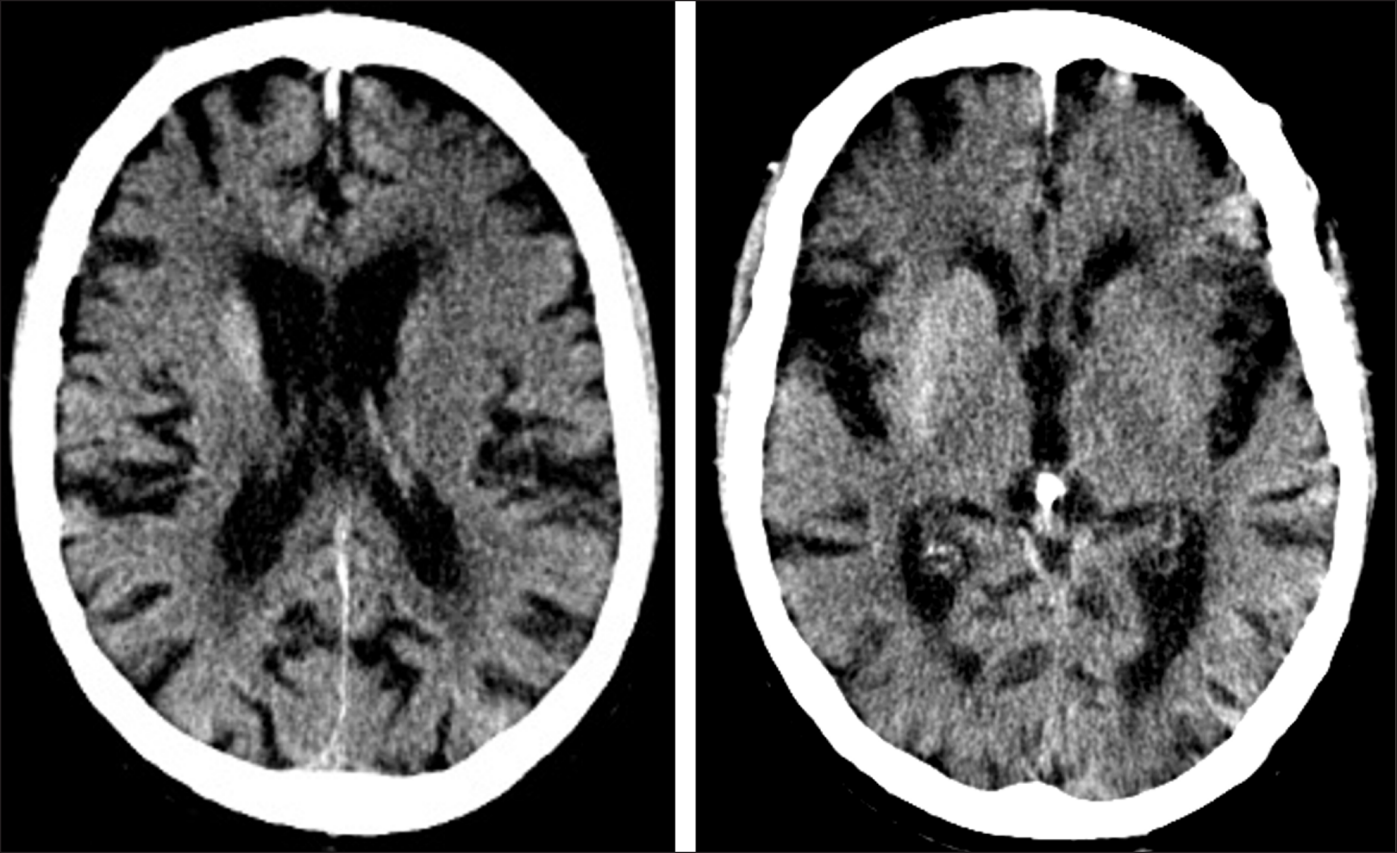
Hemoglobin A1c (HbA1c) and blood glucose level were measured. The glycemia was normal, but high HbA1c of 13.8% or 127 mmol/mol (normal 4–6% or 20–42mmol/mol) confirmed the diagnosis of new onset diabetes mellitus. During the work-up for the diabetes, abdominal CT demonstrated a mass in the body of the pancreas, believed to be the cause of the diabetes mellitus. Considering the patient’s mental state, no further investigation was performed.
